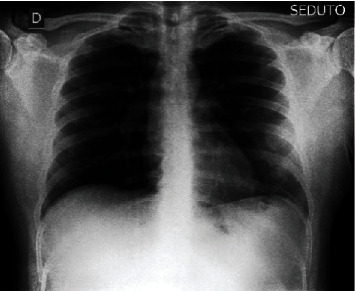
X-ray of COVID-19-affected chest.
